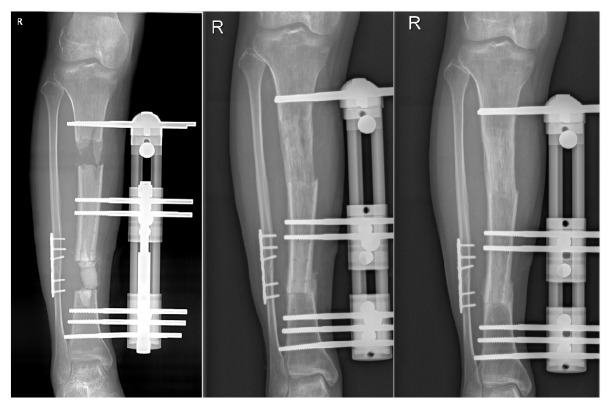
The figures in the left, middle, and right position showed the anteroposterior X-ray photographs taken about 1, 8, and 13 months after surgery.
